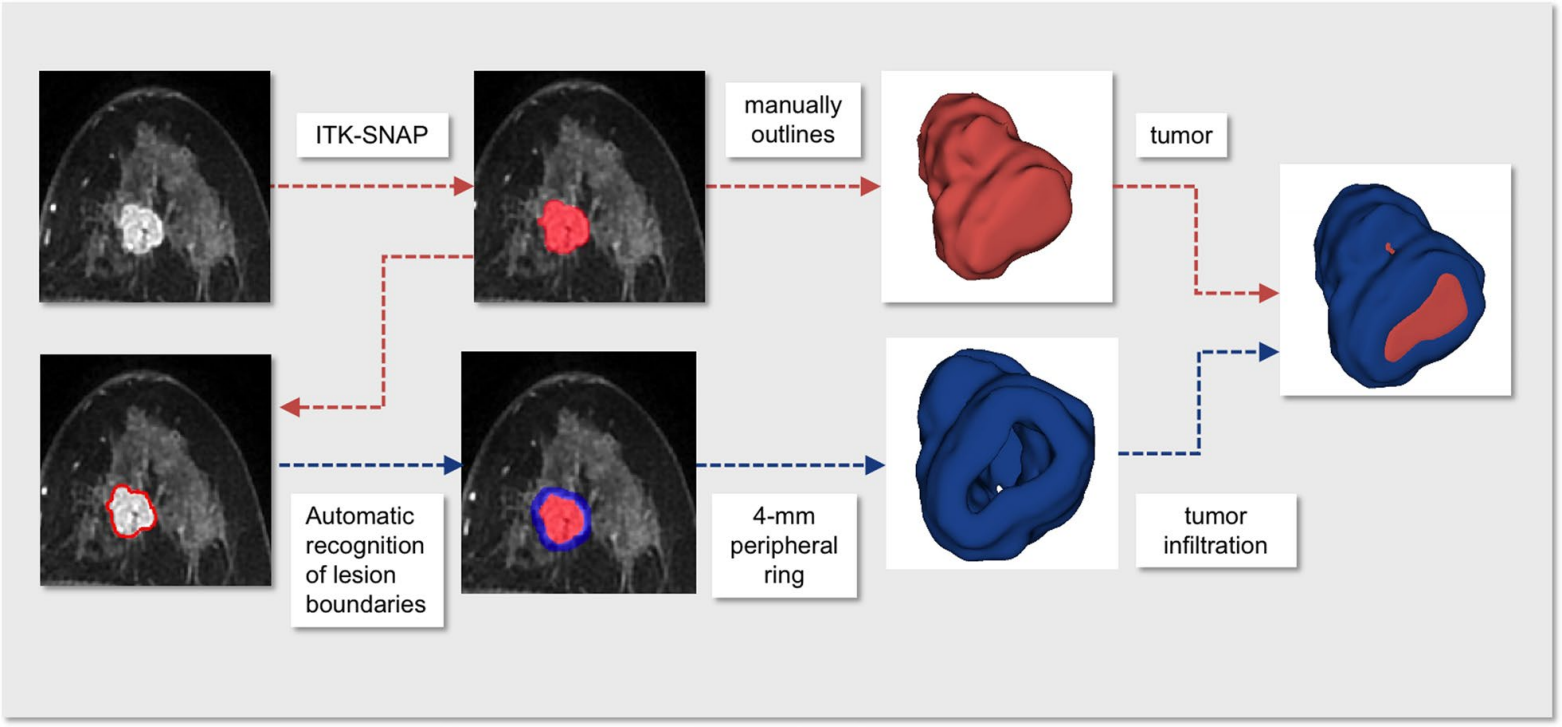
Lesion segmentation. The core tumor area in MRI imaging was measured and outlined manually by ITK-SNAP software in different slices. The boundary of the tumor was automatically identified by Python. A peripheral region was then established inside and outside 2 mm away from the defined boundary, consequently forming a 4-mm thickness ring to represent the extension of the tumor infiltration
